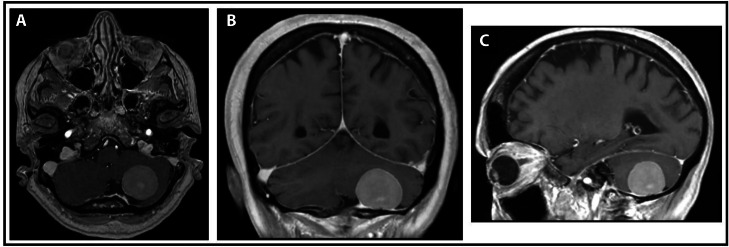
Contrast-enhanced T1-weighted brain MRI images in the axial (A), coronal (B), and sagittal (C) planes, respectively. These images show a well-defined, enhancing left cerebellar extra-axial mass consistent with a meningioma, associated with mild mass effect on the vermis while the fourth ventricle remains patent.
